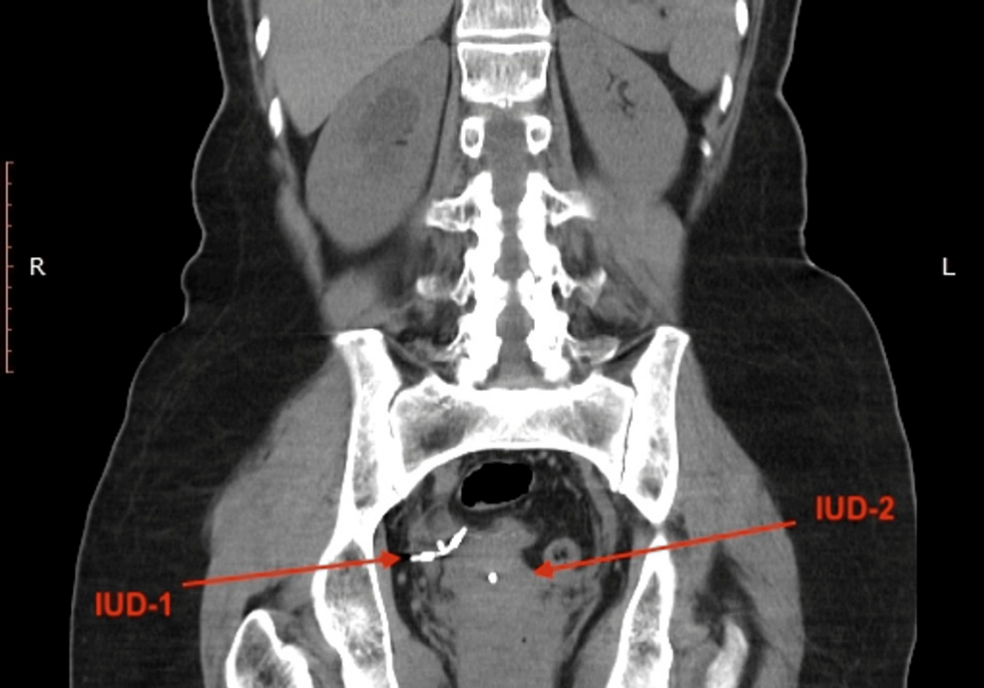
Right hydronephrosis and both IUDs were visible in the coronal planes of computed tomography. IUD: intrauterine device.
